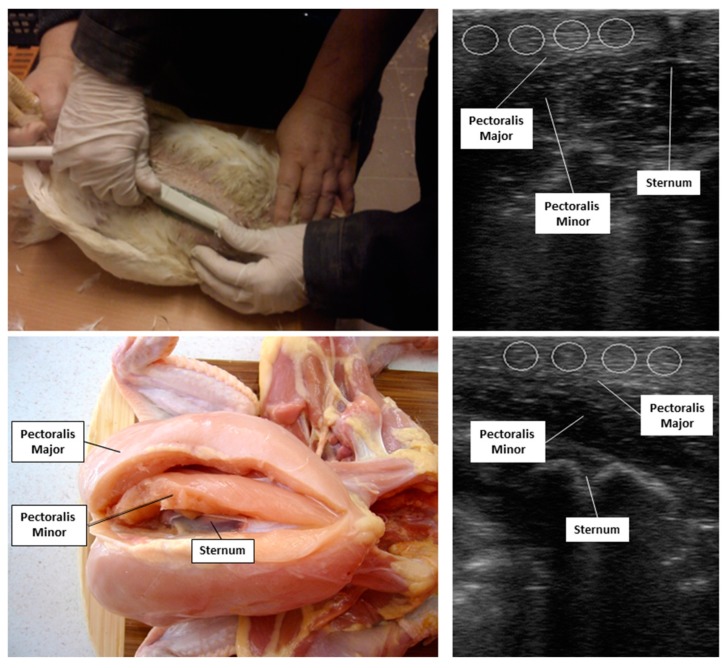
In situ ultrasonographic examinations (upper left) of pectoralis major muscles (bottom left—https://kriegerscience.wordpress.com/2010/11/09/dissecting-a-whole-chicken/) in broiler chickens (n = 40) scanned in a transverse (upper right) or longitudinal (bottom right) plane: The white circles in the panels depicting ultrasonograms indicate the potential placement of four computer-generated circular areas of interest (spot meters) used for echotextural analyses of the muscle tissue.
